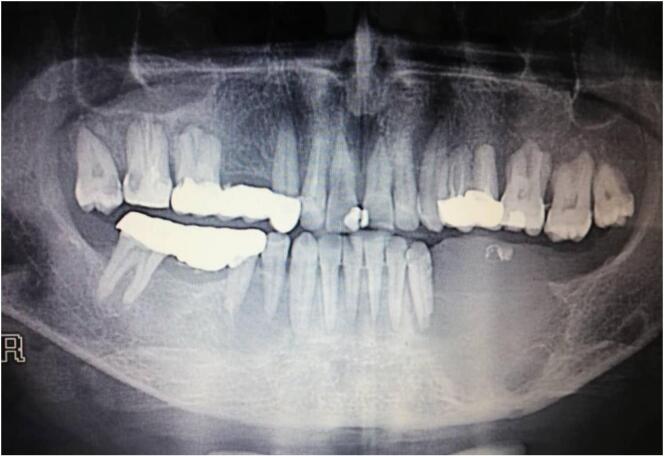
Huge corticated radiolucency with well-defined borders measuring 3 × 4 cm in panoramic radiograph.
